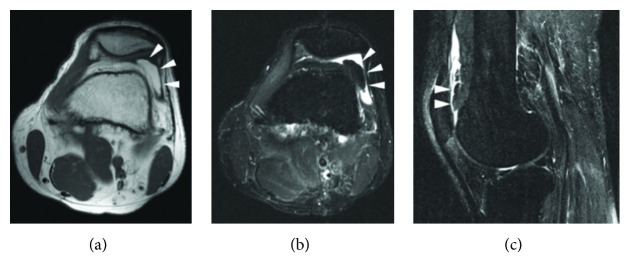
Preoperative MRI. Axial T1 MRI (a), axial STIR MRI (b), and sagittal STIR MRI (c) revealed the prefemoral fat pad (white arrowhead). The fat pad was located superolaterally to the PF joint, and hyperplasia was confirmed. There were no fibrous septa or mild peripheral enhancement patterns that were suggestive of lipoma.
